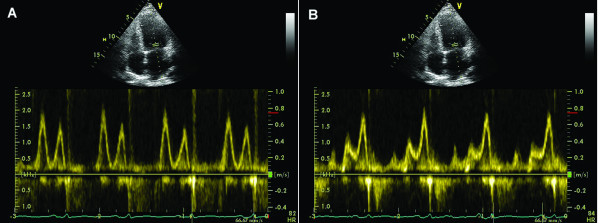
55 year-old woman, with LV hypertrophy and moderately dilated left atrium. TTE, apical 4C view, mitral inflow pulse Doppler patterns. A. Mitral inflow pattern appears "normal" at rest. B. During Valsalva maneuver the E/A ratio decreased from 1.3 to 0.6 and peak A velocity increased from 60 to 75 cm/s, unmasking the impaired relaxation pattern.
